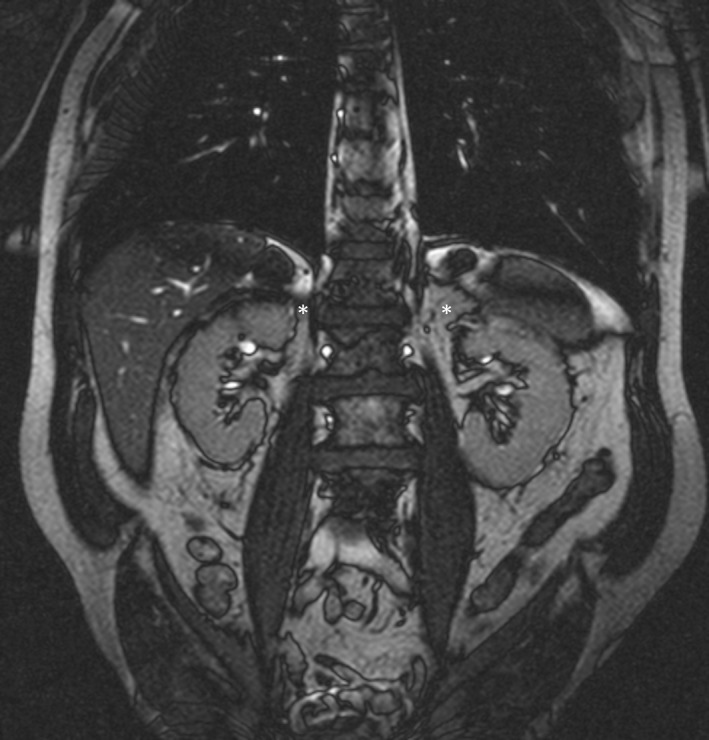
MRI patient. Abdominal MRI in the patient with glucocorticoid‐remediable aldosteronism did not show any abnormalities (adrenal glands marked with *“*
